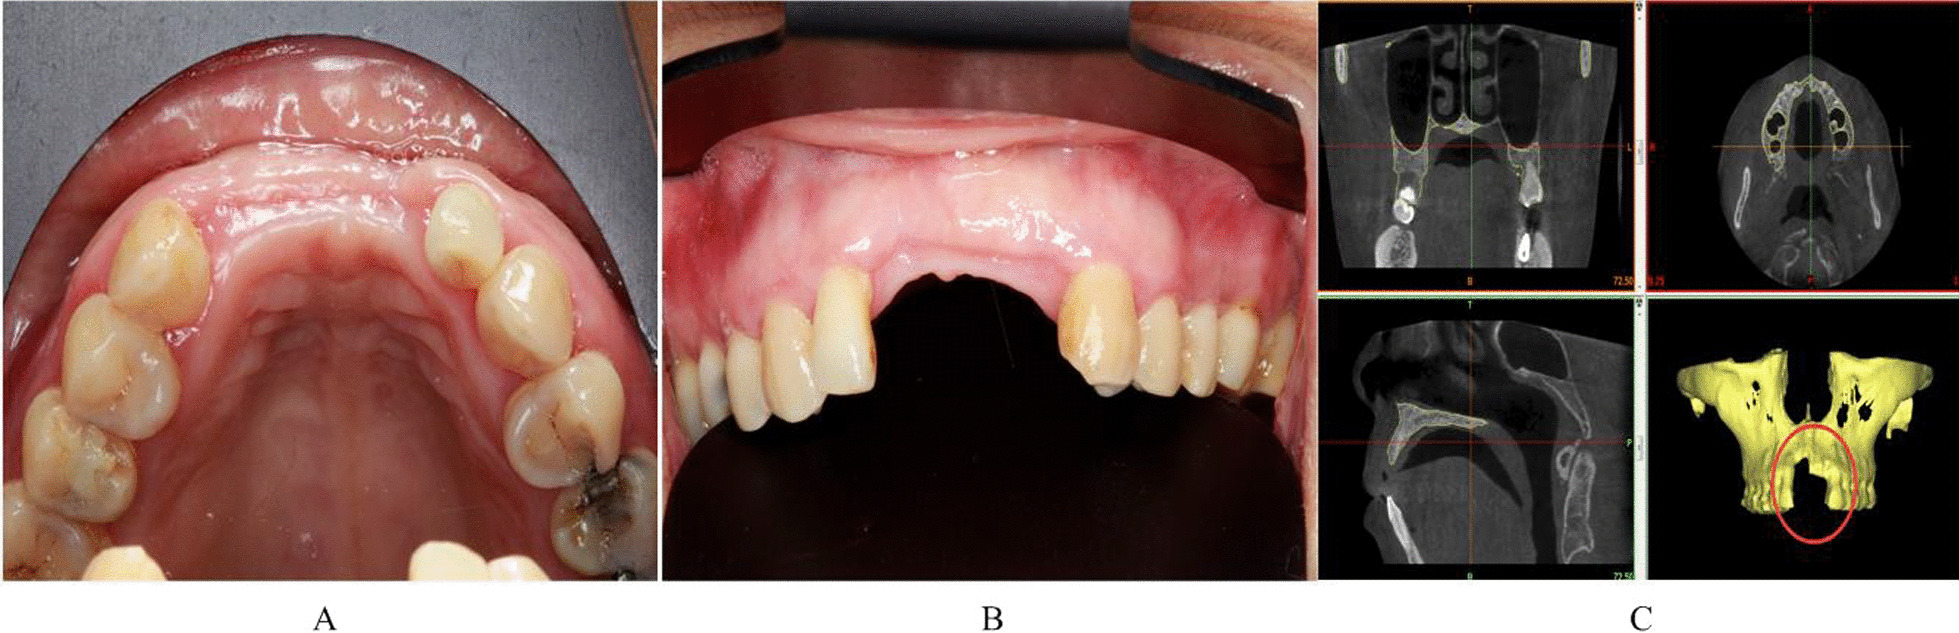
Preoperative intraoral photograph: A maxillofacial view; B front view; C CT imaging and 3D reconstruction
